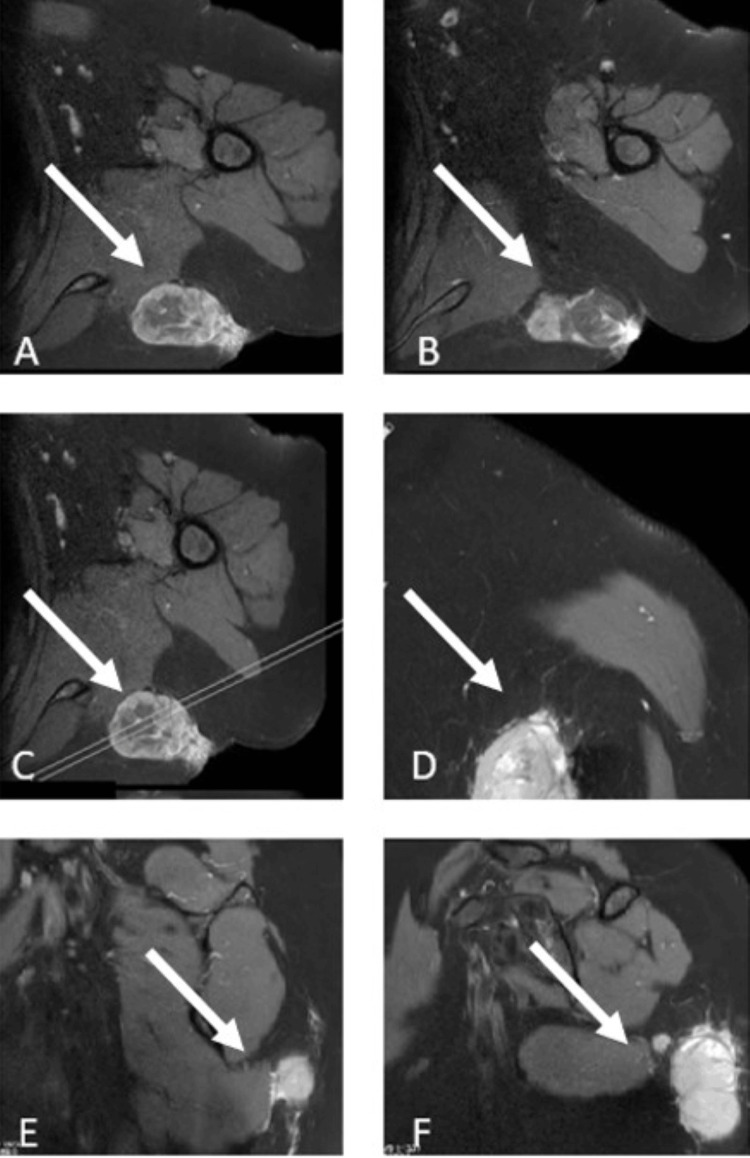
The preoperative magnetic resonance imaging (MRI) of the first patient, a 60-year-old female, demonstrates a lesion within the soft tissue of the left scapular region. The white arrows indicate the mass in the interscapular region.
